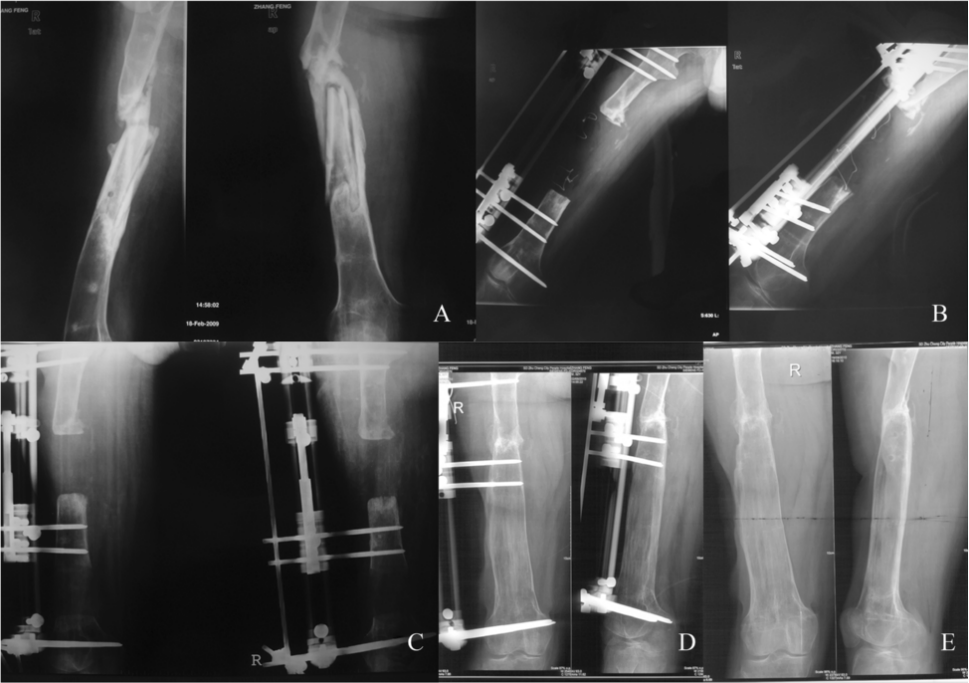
A 33-year-old man who had an infected femur nonunion. (A) Radiograph of a 33-year-old man who had an infected femur nonunion. (B) Debridement of the site of infected nonunion with 8-cm bone defects and corticotomy of femur. (C) Two months after operation with bone transport. (D) Twelve months after operation, good consolidation of the regenerate and complete bone union at the docking site was presented. (E) The external fixator was removed at 12 months after operation.
